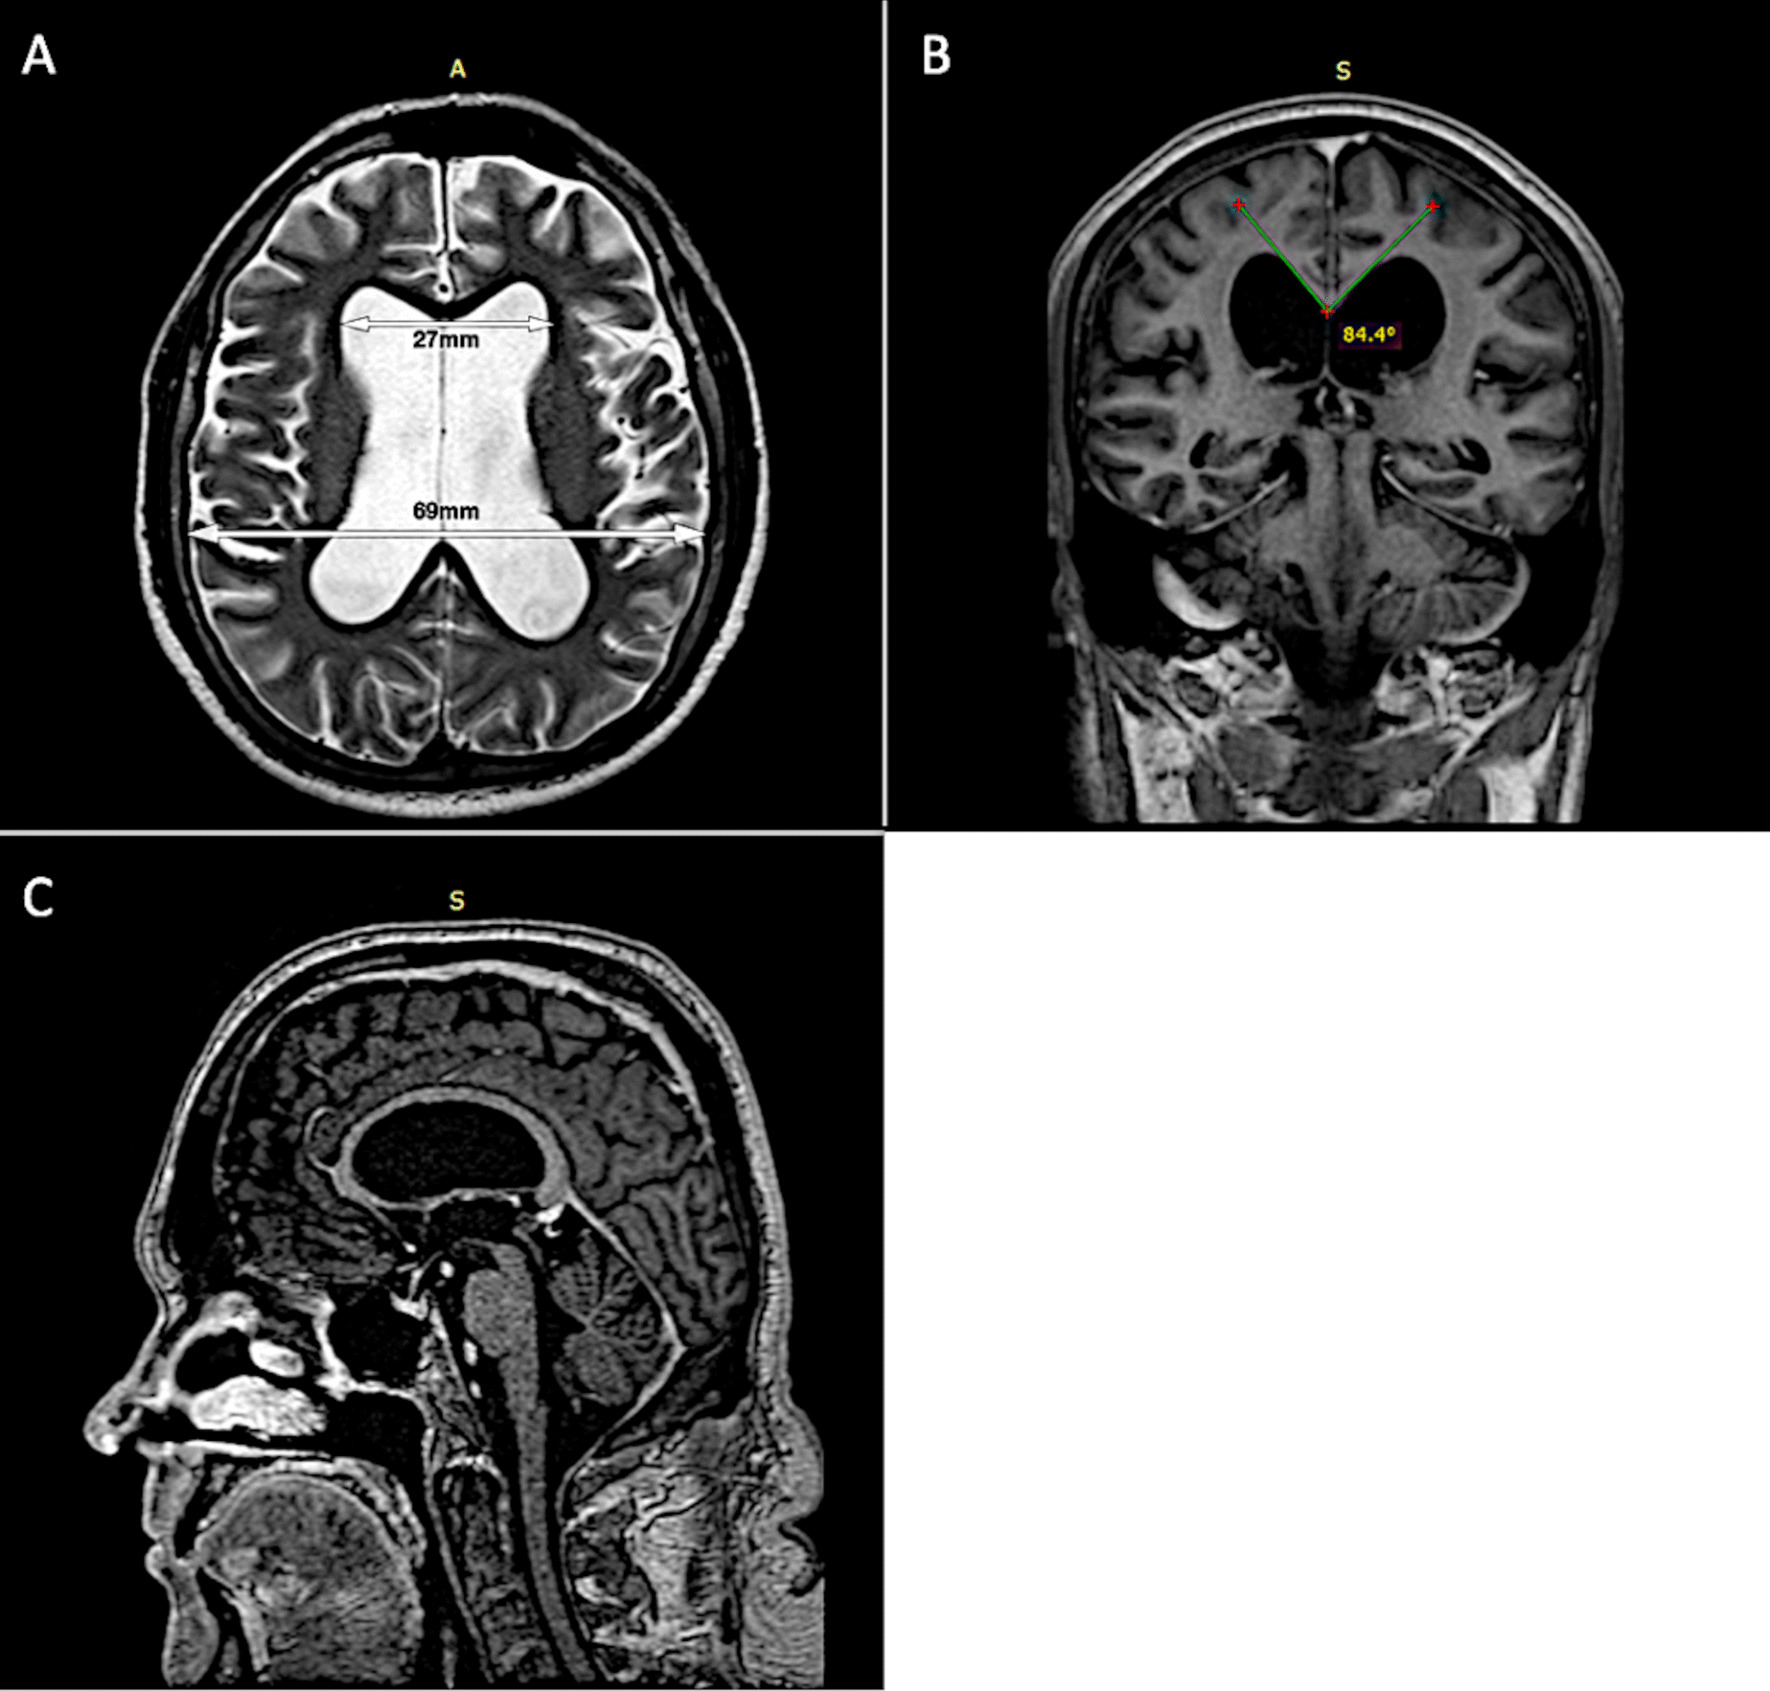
Brain MRI in a patient with normal pressure hydrocephalus after COVID19 infection showing: A Axial T2-weighted image with dilated lateral ventricles, B coronal T1-weighted image depicting acute callosal angle and upward bowing of the corpus callosum and C sagittal T1-weighted image showing normal infratentorial ventricular compartment
